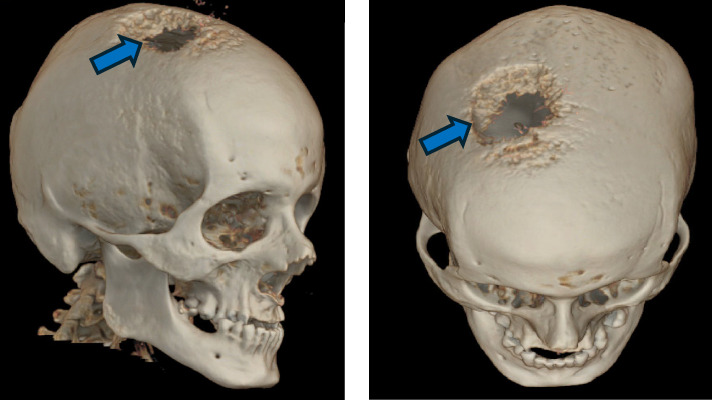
Three dimension computed tomography of the head shows a right parietal bone destruction (blue arrow) by metastasis from differentiated high grade thyroid carcinoma. The metastasis destroyed the parietal bone, invaded the dura and scalp and protruded extracranially.
